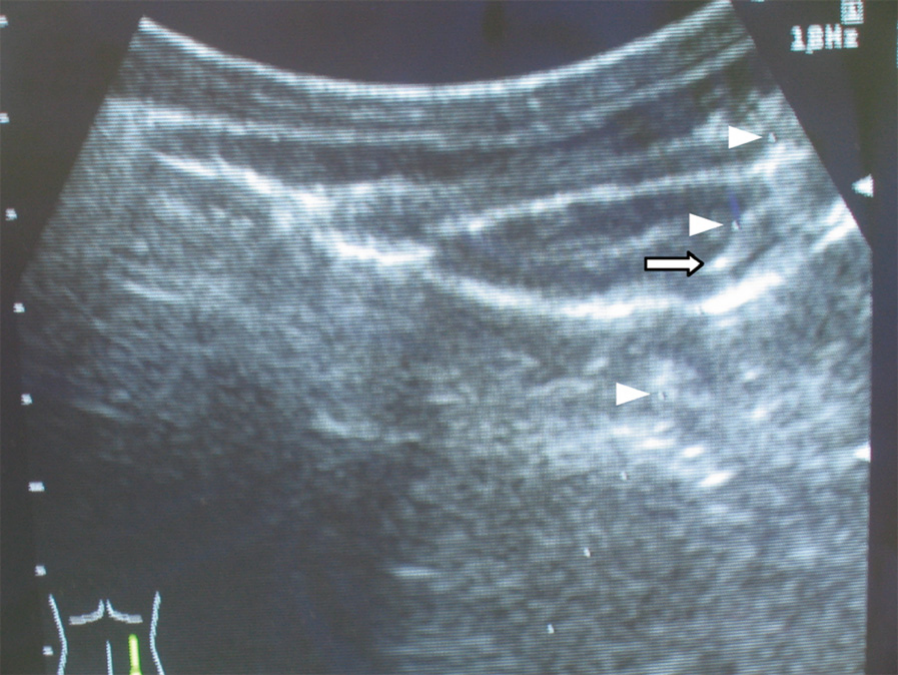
Ultrasound image of the perfused Bento kidney. Arrowheads show the progress of the biopsy needle, with the tip indicated by the arrow.
